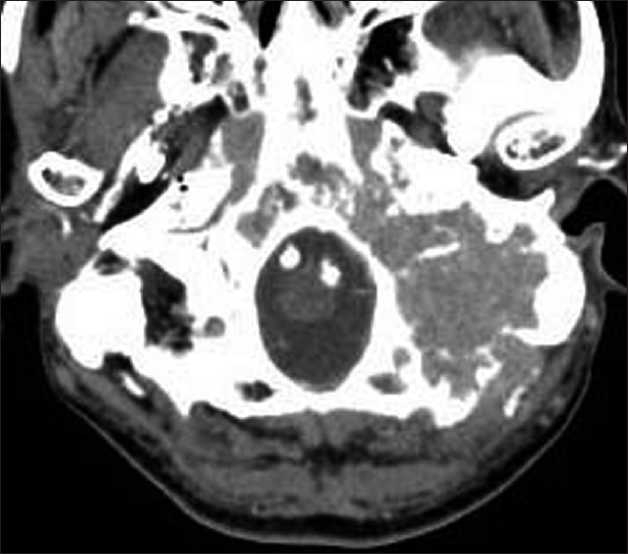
CT scan with contrast medium of the head showing a homogeneously enhanced lesion in the clivus and petrous bone with extensive osteolytic reaction
